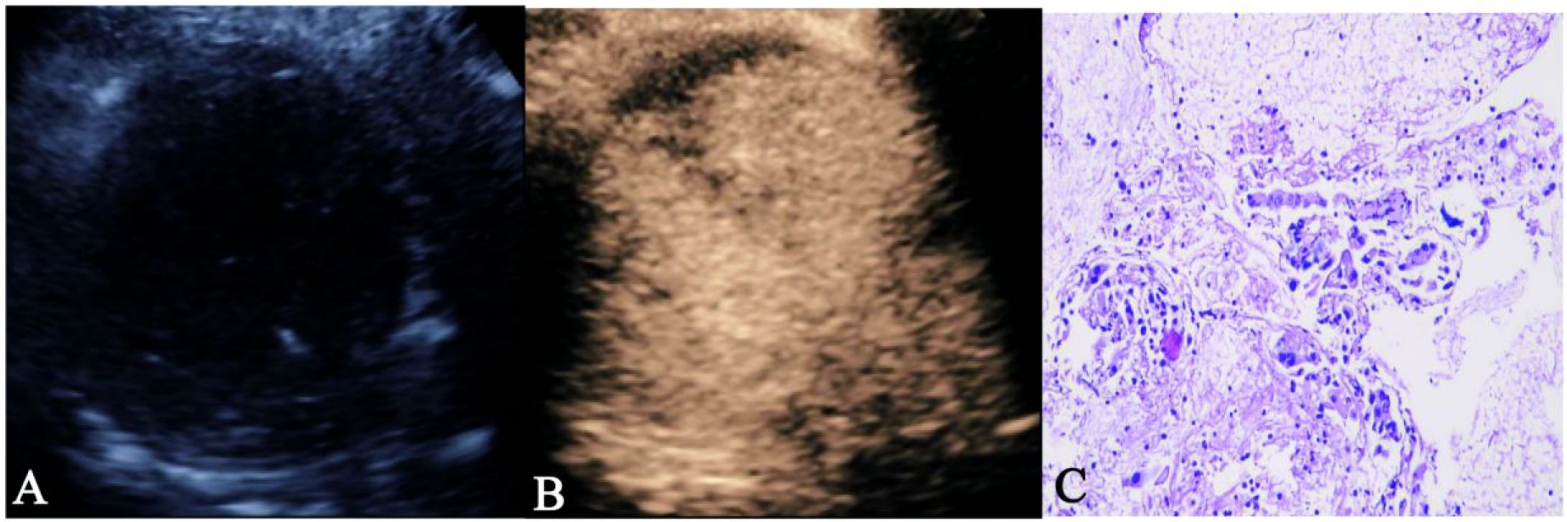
The 5A–C showed a case of 50-year-old female NSCLC with EGFR-mutant. (A) The gray-scale ultrasound shown a hypo-echoic lesion with well defined boundary and air bronchograms. (B) On the contrast-enhanced ultrasound, the lesion exhibited homogeneous hyperenhancement. (C) The pathological image confirmed the lesion as a NSCLC.
